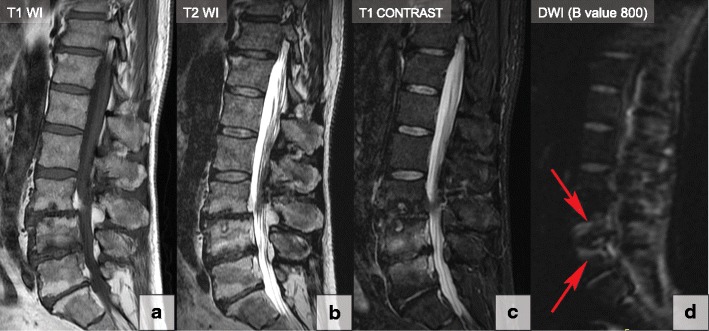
The claw sign in type 1 degenerative bone marrow (Modic) changes at L4–L5. T1-WI (a), T2-WI (b), T1-contrast-enhanced (c) and DWI sagittal images (B value 800) (d). The claw sign is identified in the DWI image as linear paired regions of high signal located within adjusted vertebral bodies at the boundaries between the normal and vascularised bone marrow (red arrows). Please note that type 2 degenerative bone marrow changes at L5–S1 and L3–L4 do not demonstrate the claw sign
